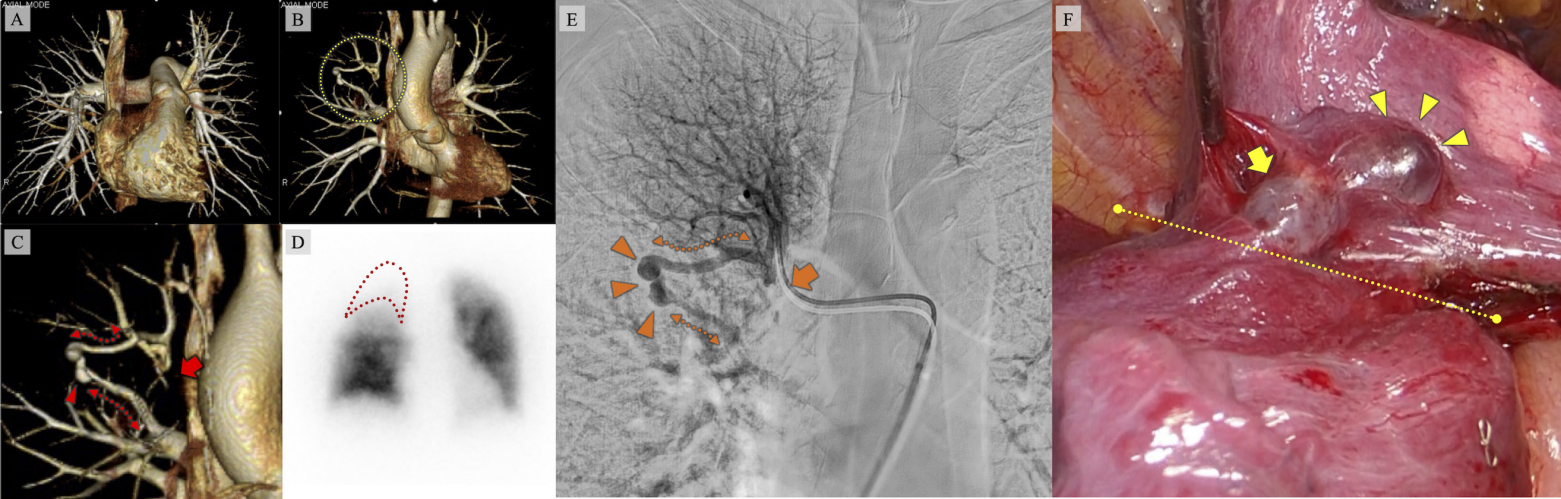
(A) No abnormal vessels detected in the pulmonary arterial phase of the four‐dimensional reconstructed enhanced chest computed tomography (4D‐CT). (B) In the pulmonary venous phase of 4D‐CT, abnormal vessels are visualised in the right upper lobe (dotted area). (C) In the same phase, occlusion of the right pulmonary vein (arrow) is seen, with an abnormal pulmonary vein branching upstream of the occluded segment (dotted line) and rejoining the pulmonary vein in the middle lobe via a pulmonary vein varix (triangle). (D) Pulmonary perfusion scintigraphy showing no abnormal hyperaccumulation in organs outside the lungs, with decreased blood flow in the right upper lobe (dotted area). (E) Selective pulmonary arteriography of the right upper lobe revealing right pulmonary vein occlusion (arrow) with pulmonary venous varix (triangle) and abnormal vessels (dotted line). (F) Intraoperative view showing the pulmonary venous varix along the interlobar fissure (triangles) and a cross‐fissural venous channel (arrow); the interlobar fissure is indicated by the dotted line.
